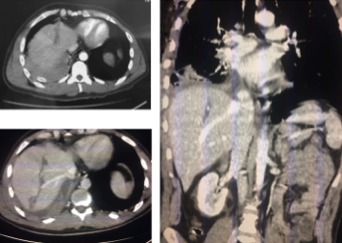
Images of abdominal CT showing the path of the chest tube thru the liver next to hepatic right and middle hepatic vein.
